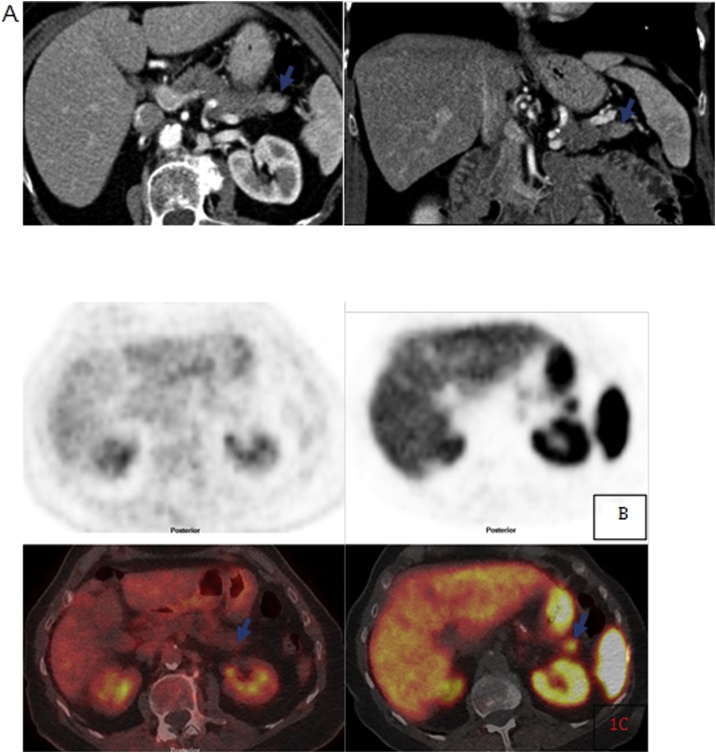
(A) Axial and coronal CT arterial phase images show an arterially enhancing intraparenchymal pancreatic tail lesion (arrows) (B) and (C) Axial PET and PET/CT images FDG (1b) show no uptake in the pancreatic tail lesion whilst the DOTATATE (1c) images show increased somatostatin receptor expression in the pancreatic tail lesion (arrows).
